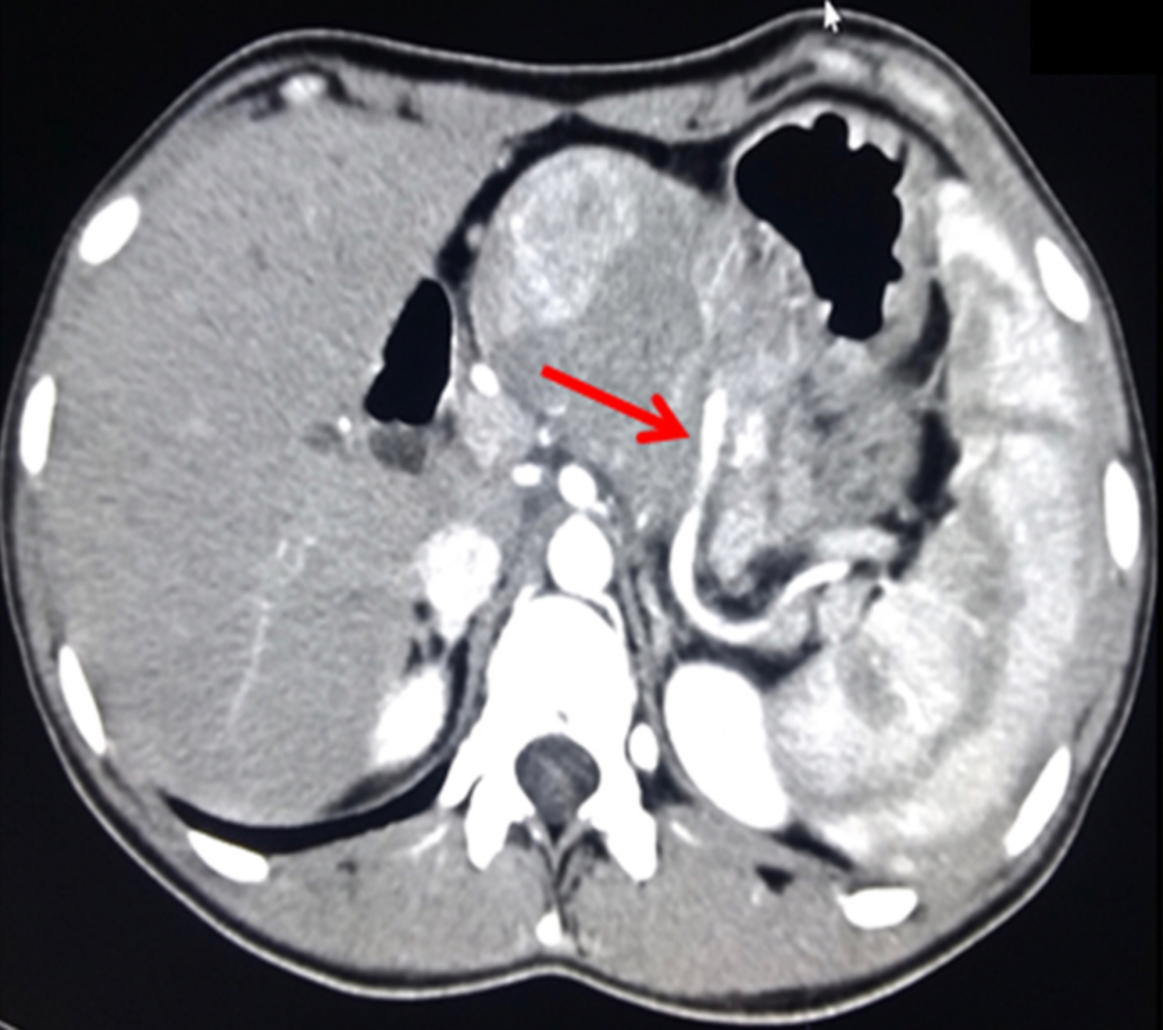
Pre-operative contrast-enhanced computed tomography (CECT) imaging of the tumor showing a solid cystic exophytic lesion from the body of pancreas with the splenic artery coursing through the tumor (red arrow).
